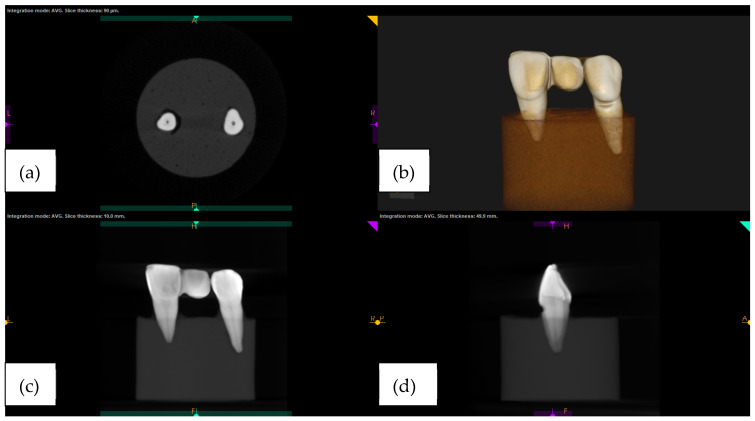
Cone beam computed tomography (CS 3D imaging) of (a) axial view; (b) 3D image; (c) coronal view; (d) sagittal view.
